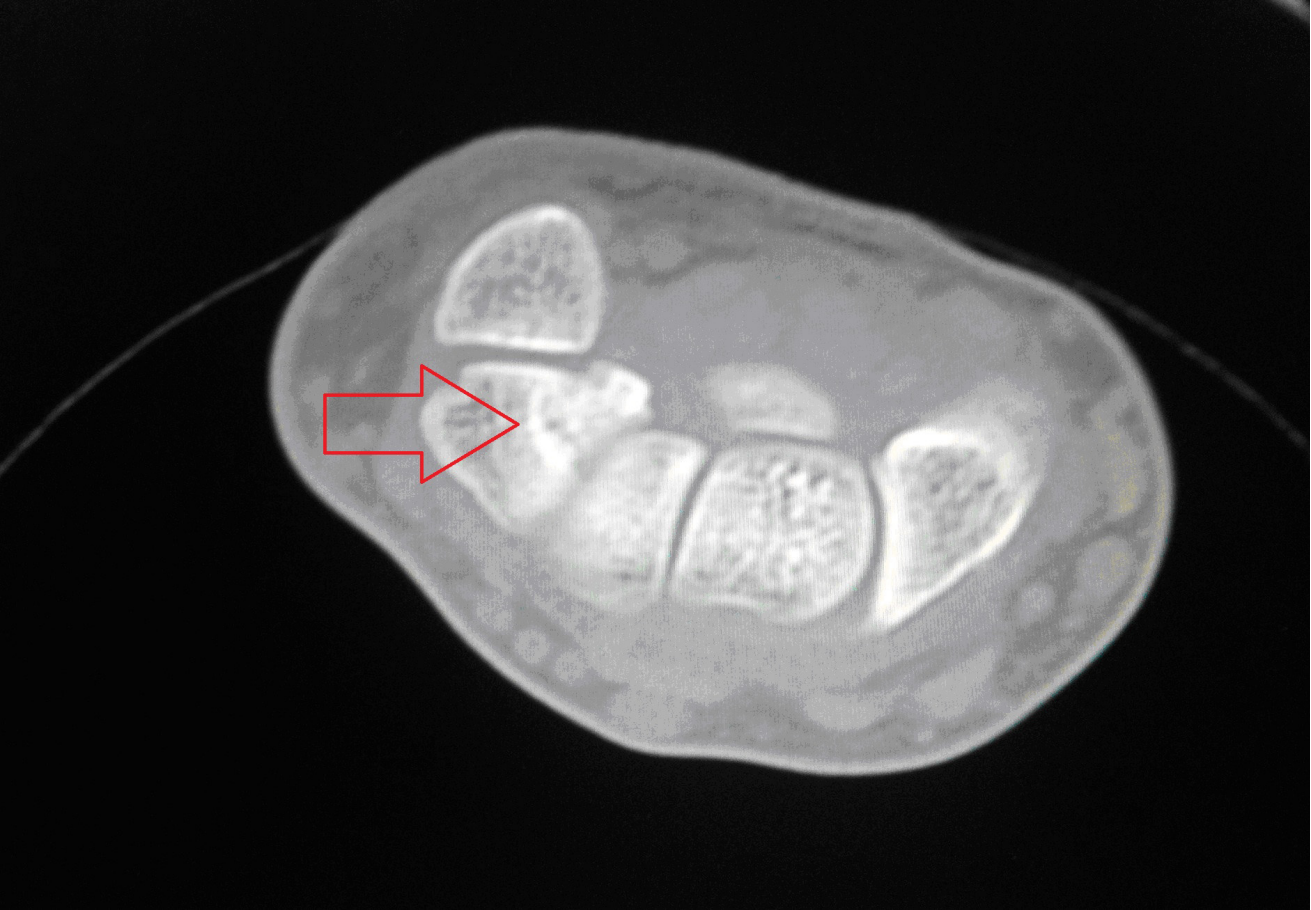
Axial computerized tomography (CT) at six months after the injury. The image shows a non-united, displaced, and isolated fracture of the body of the triquetrum.
